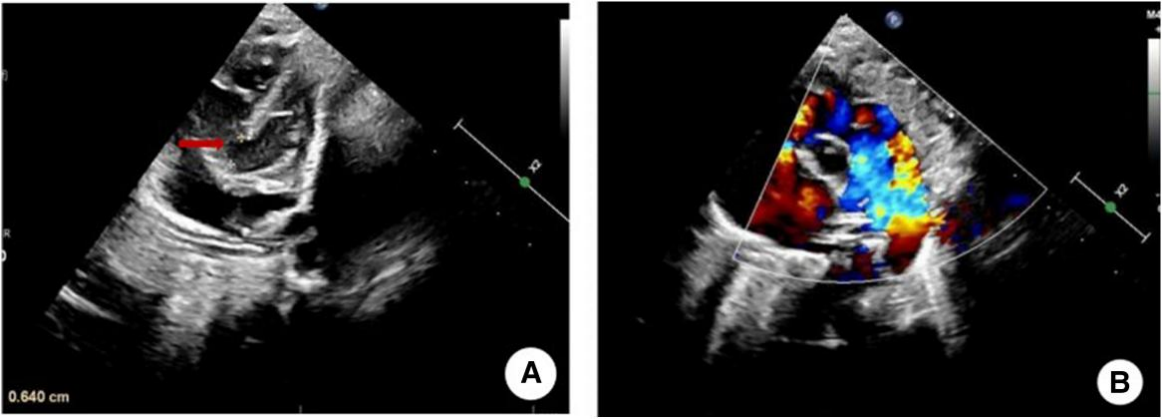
Echocardiogram. (*A*) Red arrow indicates the location of the ventricular septal defect. (*B*) Blood flow showing left-to-right shunt.
